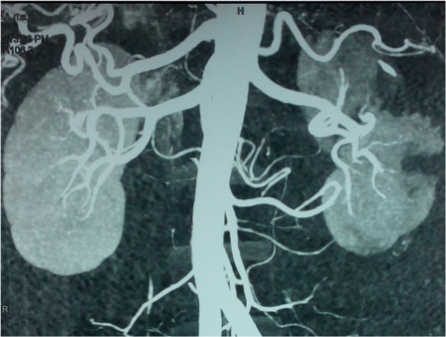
Computed tomography angiogram of abdomen revealing multiple wedge-shaped infarcts in left renal parenchyma with occlusion in interlobar branch of left renal artery supplying the mid-pole
